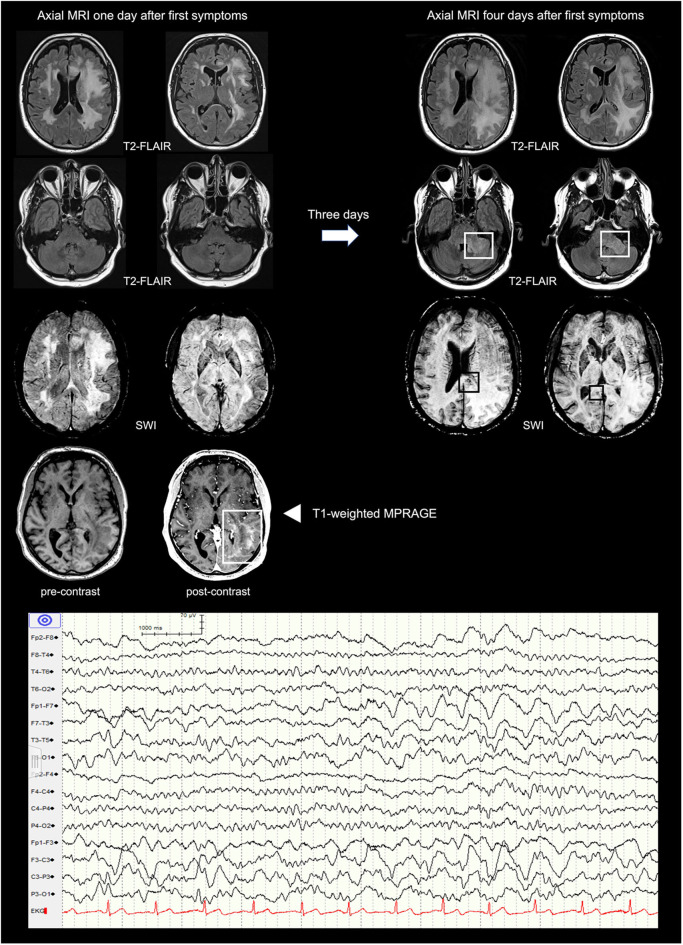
Cerebral MRI presenting the temporal evolution within 3 days (from left to right) and EEG excerpt. First row: axial T2-weighted FLAIR images showing increasing bilateral confluent widespread hyperintensities of the supratentorial white matter predominantly on the left. Second row: axial T2-weighted FLAIR images revealing new hyperintensities of the left cerebellar peduncle. Third row: axial SWI demonstrating subtle and small susceptibility artifacts in the splenium of the corpus callosum. Fourth row: axial pre- and post-contrast T1-weighted MPRAGE showing enhancement of the left parieto-occipital region. FLAIR, Fluid-Attenuated Inversion Recovery; SWI, Susceptibility Weighted Imaging; MPRAGE, Magnetization-Prepared Rapid Acquisition with Gradient Echo.
